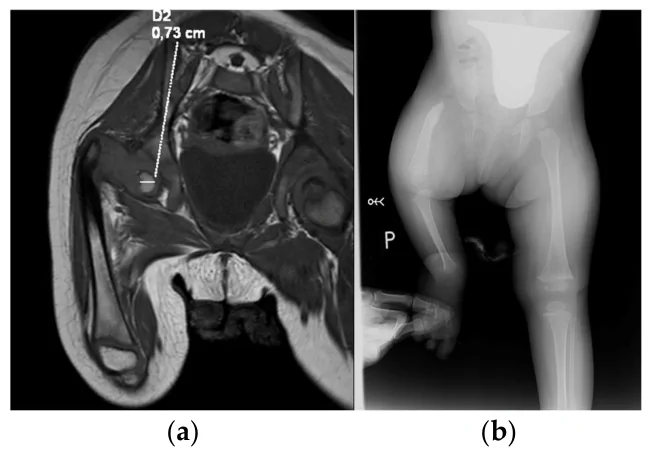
(a) MRI assessment of the hip demonstrating detailed visualization of the cartilaginous femoral head and assessment of hip stability compared with (b) plain radiography. D2—distance, P—right, figure o<-< means—supine position.
